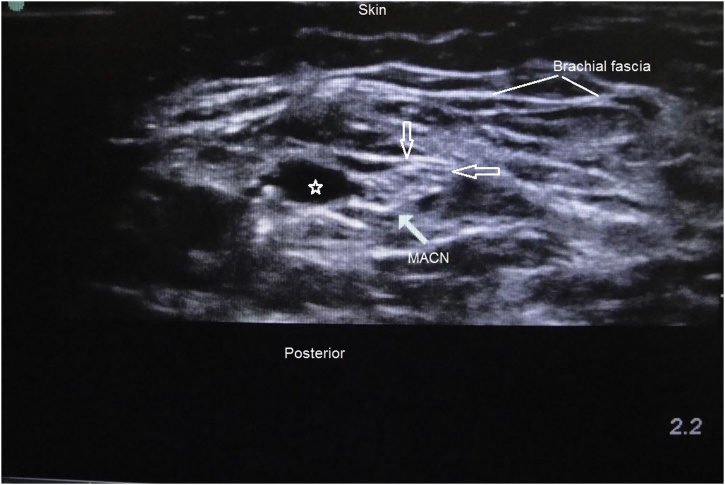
Cross-sectional sonographic view of the arm. Arrows point to the medial antebrachial cutaneous nerve (MACN) proximally. The star is at the nerve just distal to the entrapment site; showing the abnormal hyo-echoic surrounding indicating perineural edema.
